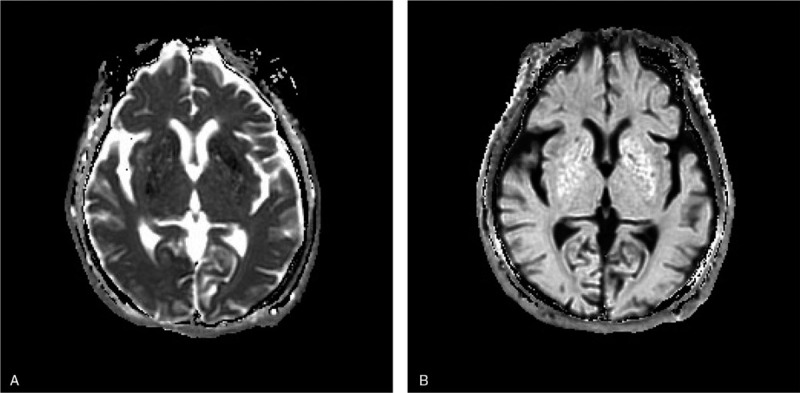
Brain magnetic resonance image after treatment: the low signals of ADC(3A) and E-ADC(3B) signal was improved than before.
